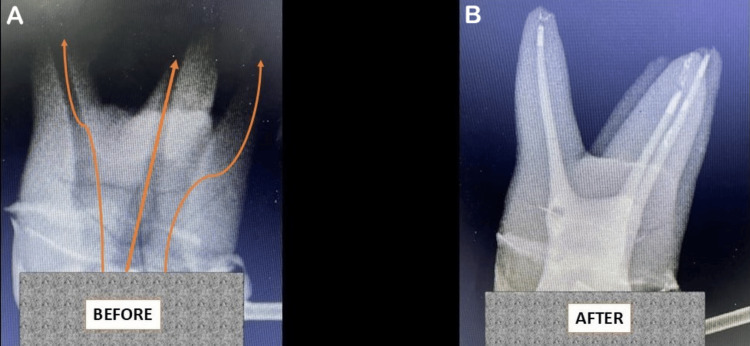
Representative radiographic images of a maxillary first molar before and after in vitro administration of a radiopaque solution using the maxillofacial drug delivery technology (A) Pre-administration radiograph demonstrating baseline anatomy of the pulp cavity of the maxillary first molar, arrows indicate the drug exit route from the pulp cavity. (B) Post-administration radiograph showing radiopaque root canals, demonstrating the path of flow.
